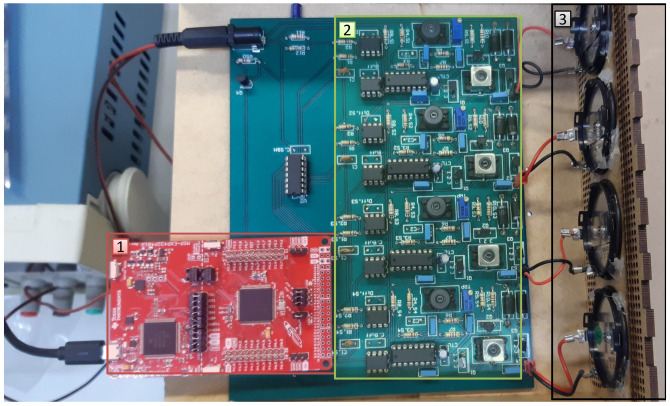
The hardware module designed for ultrasonic wave transmission and reception.
